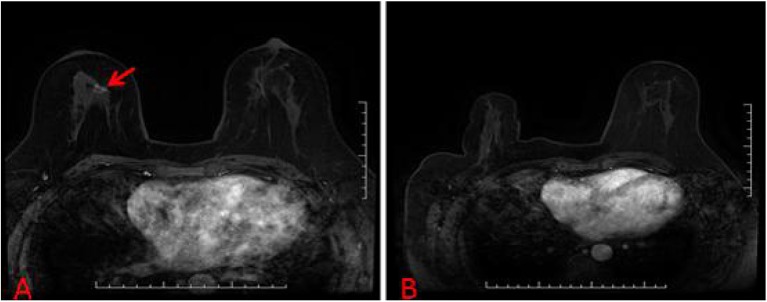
(A) A suspicious breast lesions (red arrow) that are visible only on MRI. (B) The patient with unsuccessful localization attempts because of a lack of visualization of the initial biopsy target on the scheduled day of biopsy.
